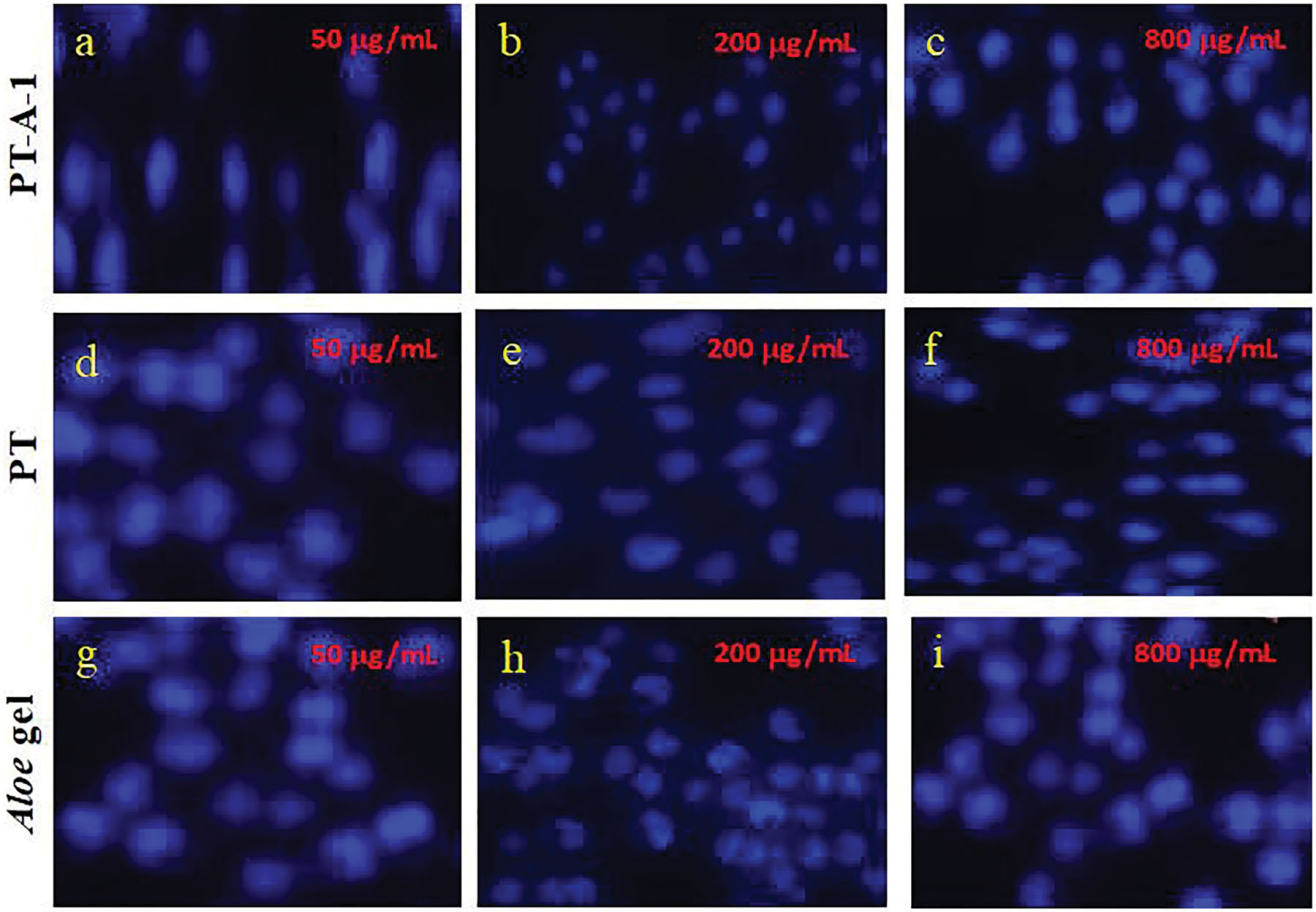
Hoechst 33258 fluorescent dyeing of HaCat cells pre-treated with different concentrations of PT-A, PT, and *Aloe* gel materials for 12 h incubation after 8 Gy X-ray irradiation (200×).
